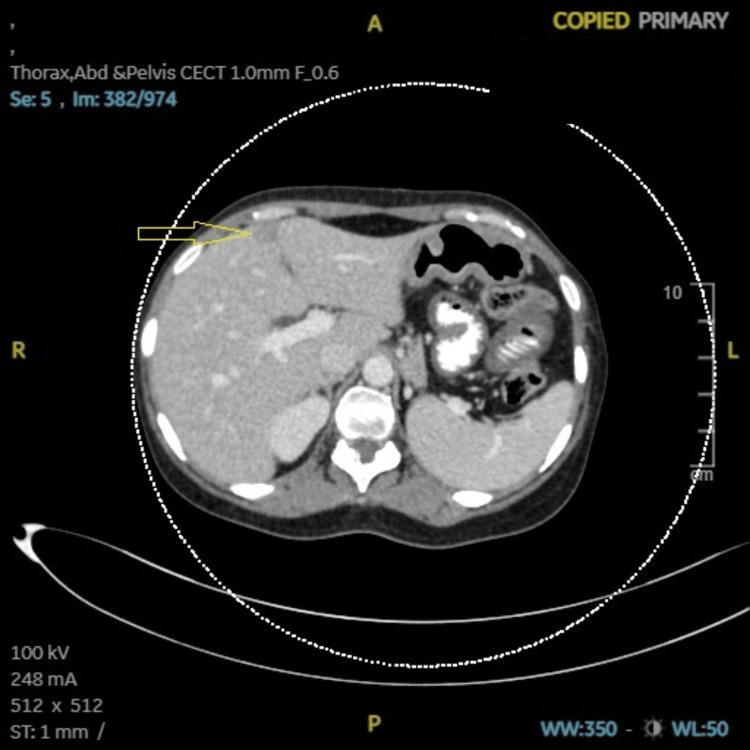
Case 1: CECT (abdomen and pelvis) showing hypoenhancing lesion (14 × 10 mm) in liver in sub-capsular parenchyma of segment IVB (arrow). CECT: contrast-enhanced computed tomography, A: anterior, R: right, L: left, P: posterior, ST: soft tissue, WW: window width, WL: window level, IVB: segment IVB of liver.
